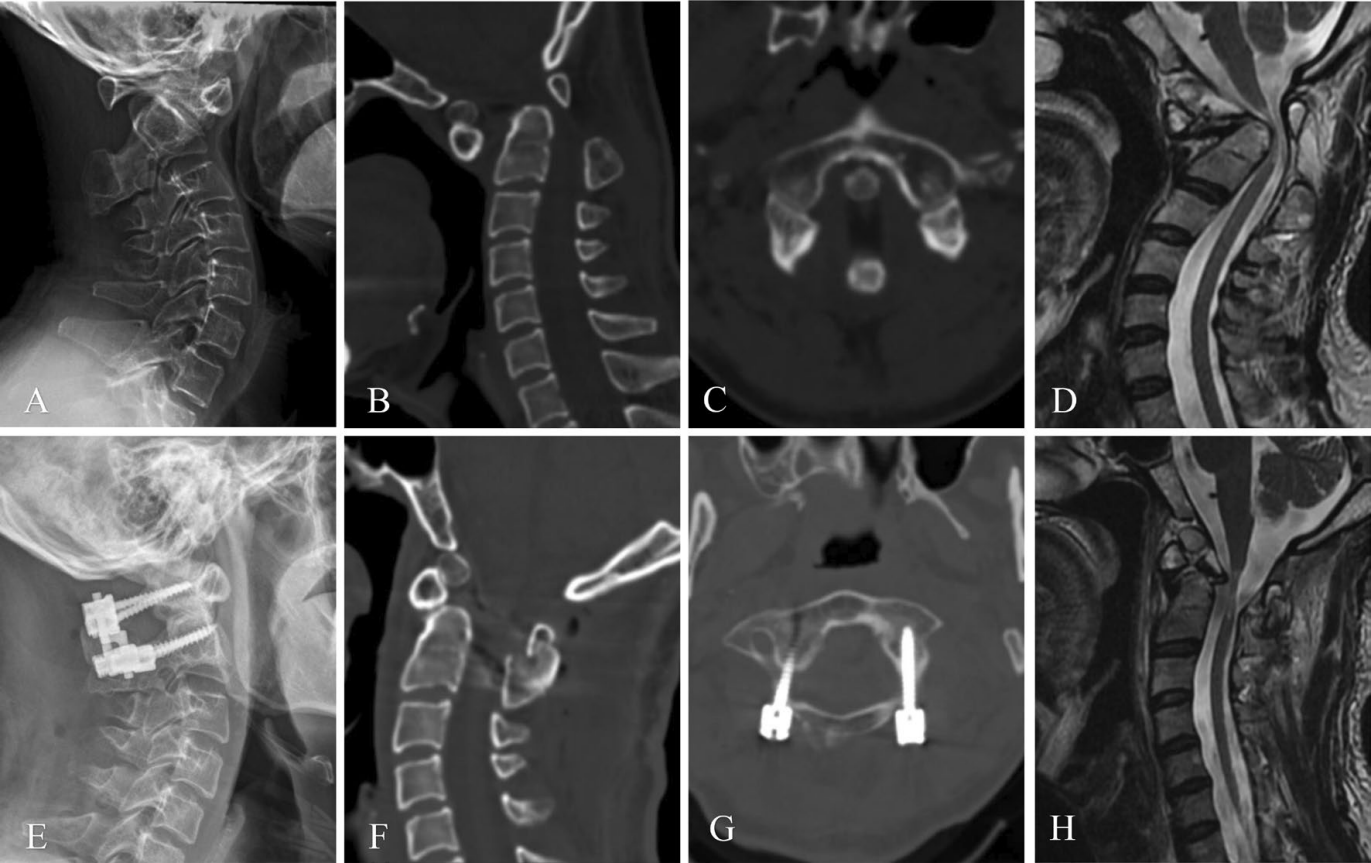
A 39‐year‐old male patient with AAD and left narrow pedicle of atlas underwent treatment with posterior unilateral (left side) C1ZAS with “in–out–in” technique combined with C2 bilateral pedicle screw fixation. (A) The lateral plain radiograph showed AAD. (B,C) Preoperative axial and sagittal CT scans showed AAD combined with Os odontoideum. (D) Preoperative sagittal T2‐weighted MRI showed decompression of the medullary and degenerative changes in C1–C2 level. (E,F) Postoperative lateral plain radiograph and lateral CT showed good reduction and position of the screws. (G) Postoperative axial plane demonstrated successful placement of the left side C1ZAS with “in–out–in” technique, without violation of the TF or dural sac. (H) Postoperative sagittal T2‐weighted MRI showed satisfactory decompression of the SC.
